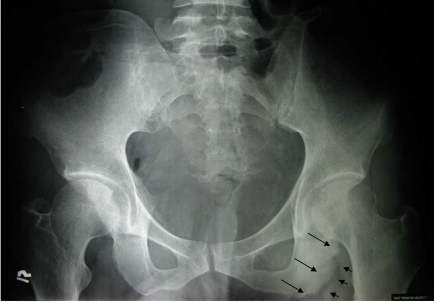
Pelvic radiograph showing area of marked sclerosis, with wide zone of transition and cortical irregularity involving the left ischial tuberosity (thin arrow). Areas of lucencies are seen in the adjacent soft tissue (arrowheads).
